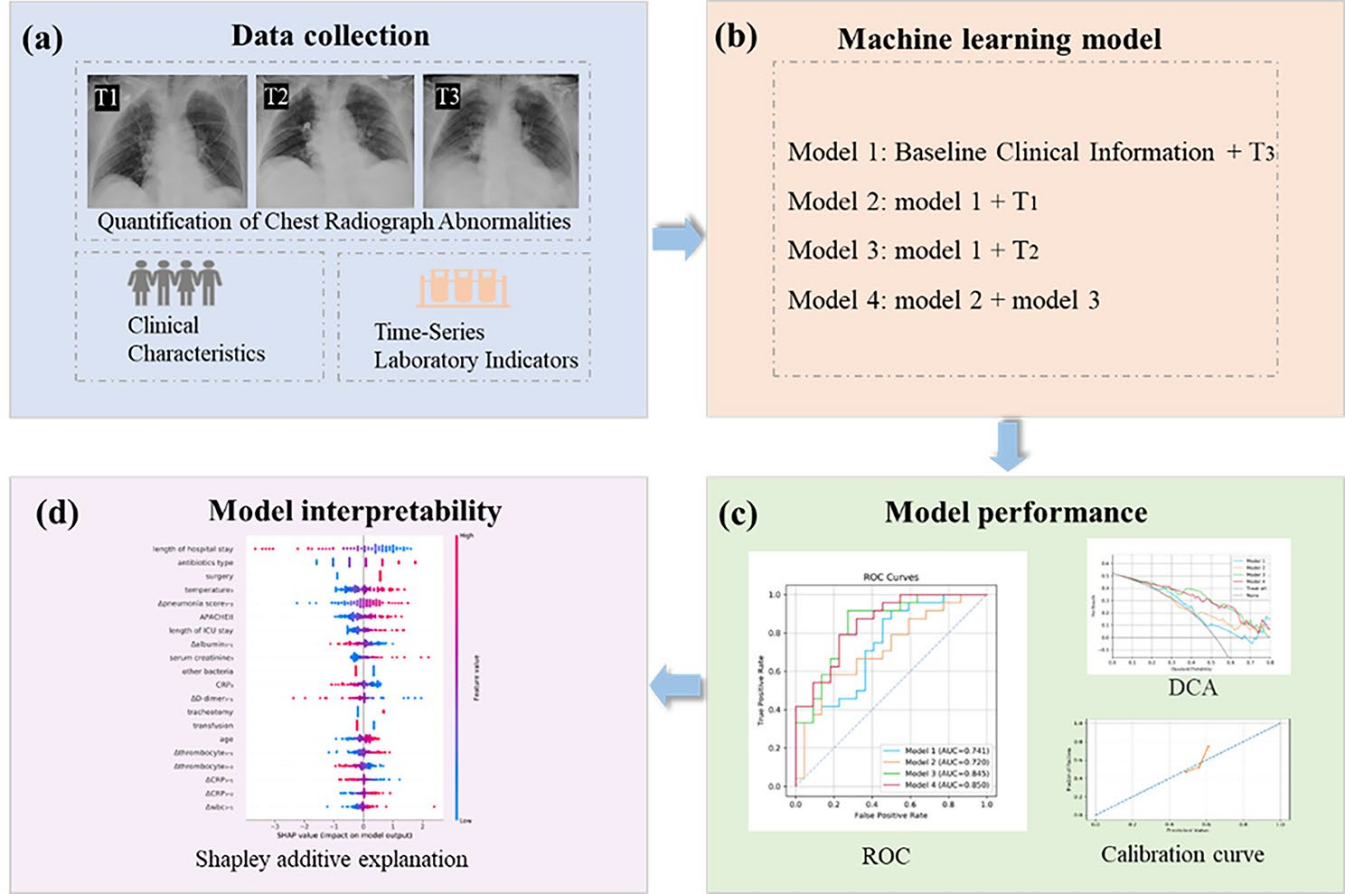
The overall design of the study. (a) Retrospective collection of baseline clinical information as well as time-series chest radiographs and laboratory indicators. Quantitative assessment of chest radiographs abnormalities, specifically through the quantitative scoring of chest radiographs by radiologists, aiming to detect and diagnose pneumonia more accurately. (b) We constructed four nested logistic regression models for classifying pulmonary infection and colonization of *A. baumannii* combined difference clinical characteristic. (c) Model performance was assessed using AUC, decision curve analysis and calibration curve. (d) We adopted Shapley additive explanation (SHAP) values to determine which features contributed most to model predictions on the logistic regression predictions. T_1_: within 1 day of admission; T_2_, 3 days before culturing out the strain; T_3_, 1 day within culturing out the strain. Model 1: clinical baseline information + laboratory indicators and radiographic features of T_3_. Model 2: model1 + the change value of between T_3_ and T_1_. Model 3: model 1 + the change value of between T_3_ and T_2_. Model 4: model 2 + model 3. ROC: receiver operating characteristic curves. DCA: decision curve analysis
